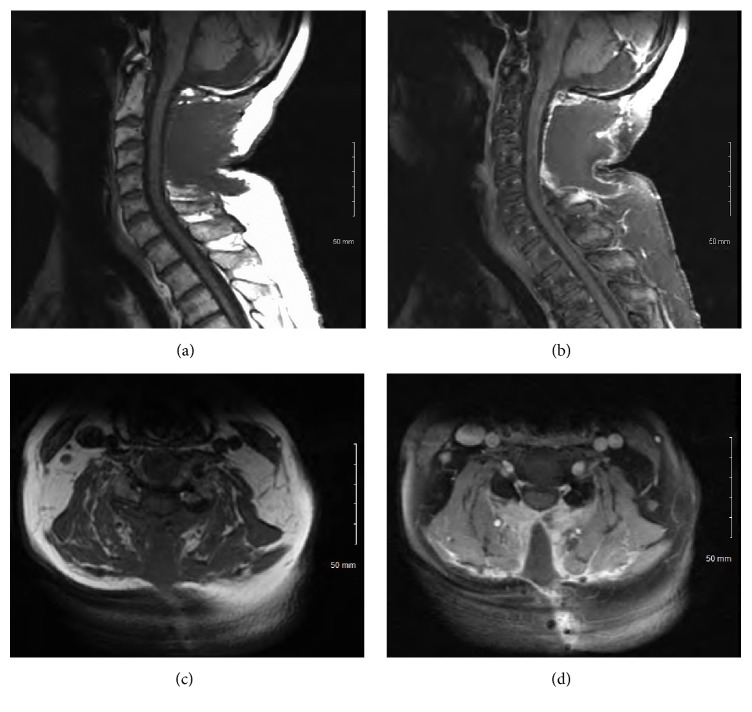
T1-sequence MRI of the cervical spine obtained 2 months postoperatively. Sagittal images without (a) and with (b) gadolinium contrast material and axial images without (c) and with (d) gadolinium, showing complete resolution of the enhancing lesion.
